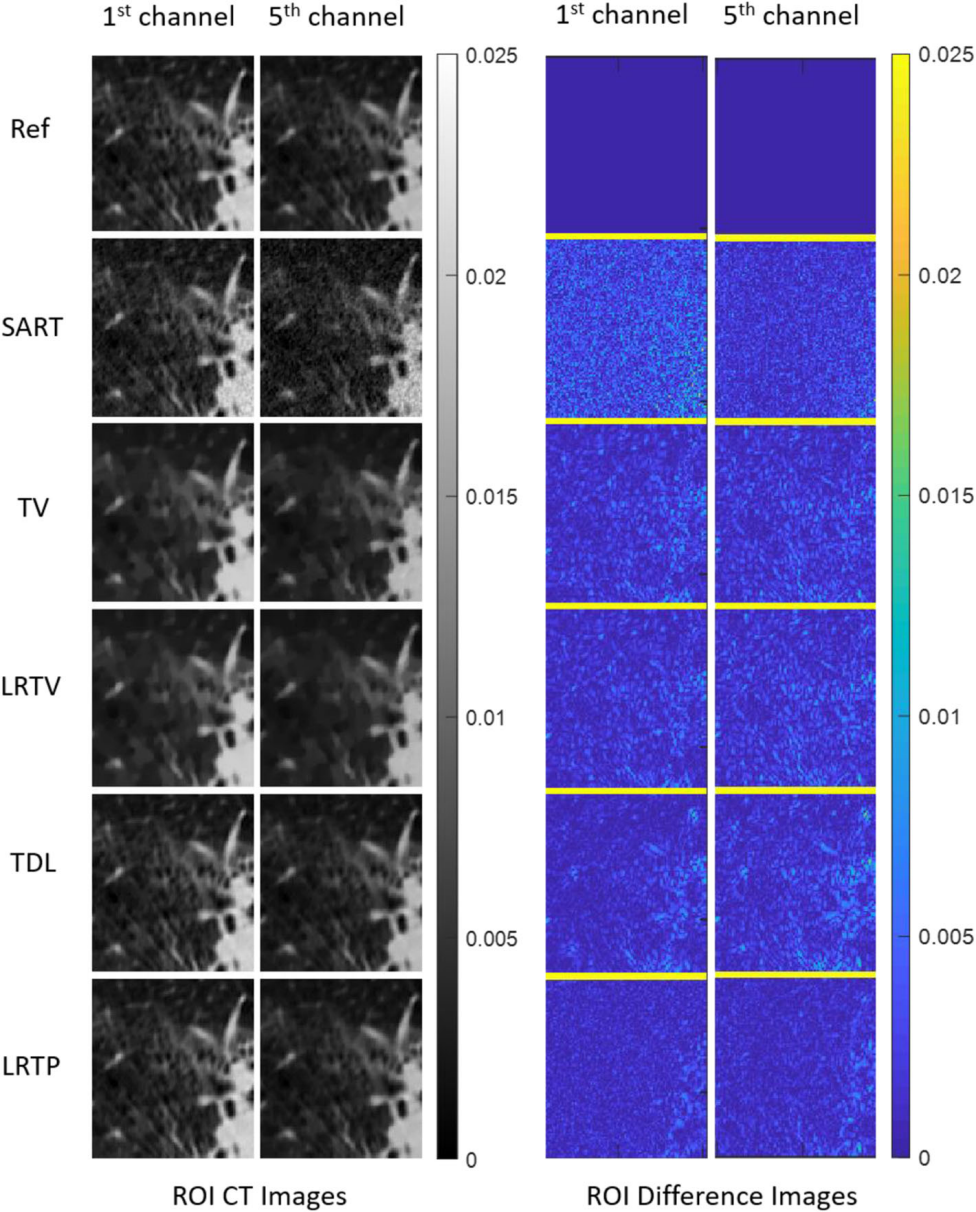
Close-up views for comparison of lung region of interests reconstructed by different algorithms. The gray level images are the magnified lung ROI CT images in the red box in Fig. 1. The corresponding absolute difference images are shown in color. From top to bottom are the reference images reconstructed from full-dose projections by SART and the low-dose images reconstructed by SART, TV, LRTV, TDL and LRTP methods, respectively. *ROI* Region of interest; *CT* Computed tomography; *TV* Total variation; * LRTV* Low rand total variation; *TDL*Tensor dictionary learning; *SART* Simultaneous algebraic reconstruction technique; *LRTP* Low rank texture prior
